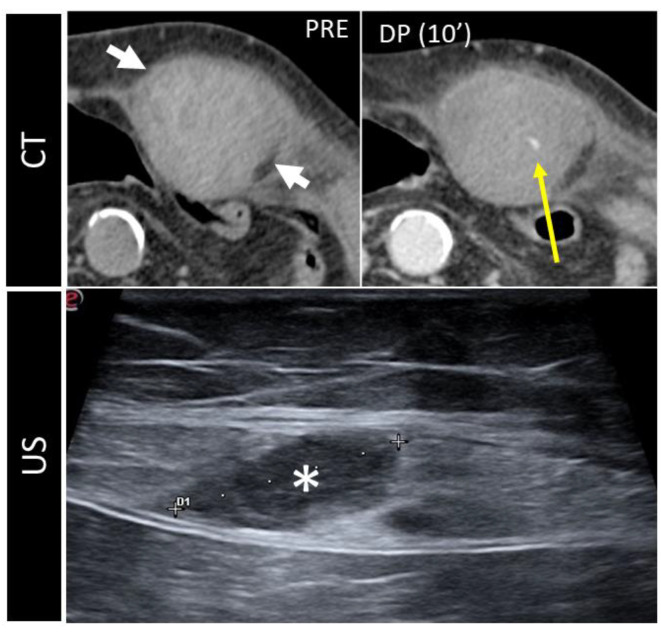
CT and US post-traumatic rectus sheath hematoma above the arcuate line. CT scan shows a well-defined mass, hyperintense on basal scan, in the context of the left rectus sheath (short white arrows). In the second picture, the delayed phase acquired 10 min after the injection of intravenous contrast demonstrates active bleeding (yellow arrow). The third picture shows the same lesion (*), studied some days later on US.
